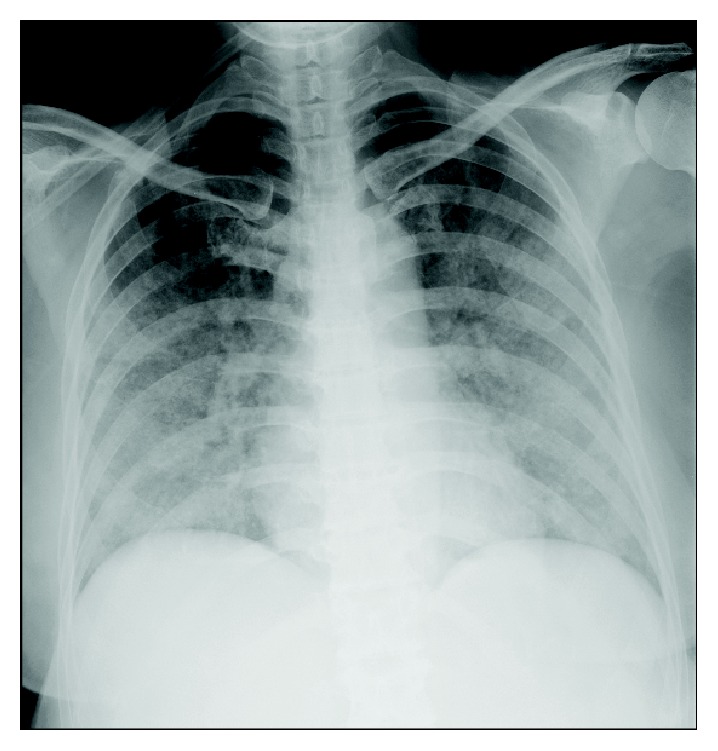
Chest radiograph showing infiltrates bilaterally.
